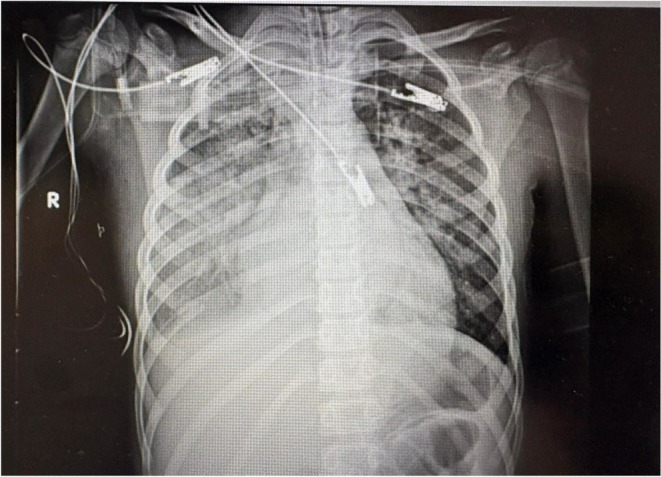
Chest X‐ray after development of respiratory distress showing bilateral pulmonary infiltrates, more pronounced on the right. Portable anteroposterior chest radiograph obtained after acute respiratory deterioration demonstrating bilateral patchy air‐space/interstitial opacities, greater in the right lung, particularly in the right upper and mid‐lung zones, with milder left perihilar and lower‐zone opacities. The findings are consistent with bilateral pulmonary infiltrates/consolidative changes in the setting of worsening hypoxemia and suspected sepsis‐associated acute respiratory distress syndrome.
